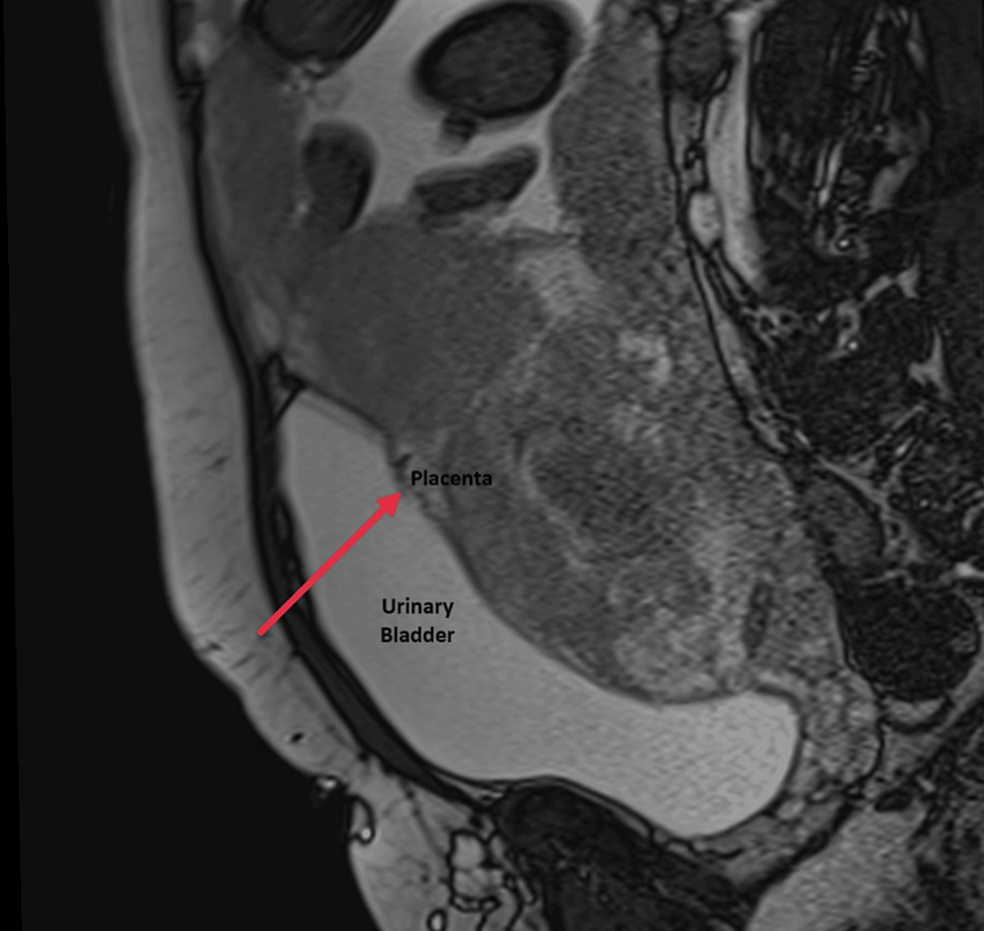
MRI scan image (sagittal view) The arrow showing the heterogenous placenta invading the posterosuperior border of the urinary bladder through the myometrium MRI: magnetic resonance imaging
